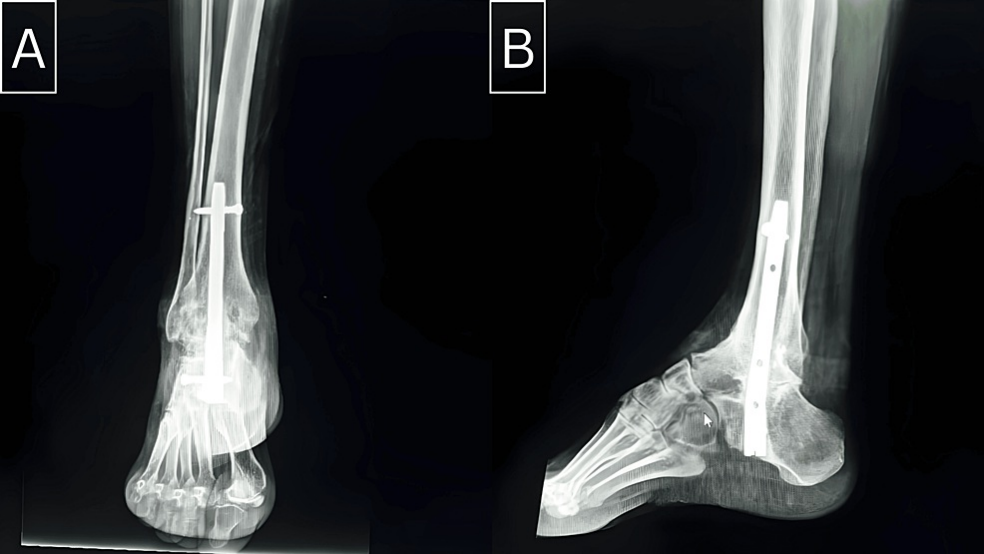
Weight-bearing anteroposterior (A) and lateral (B) X-ray of the ankle five months after tibio-talo-calcaneal arthrodesis with a retrograde femoral nail.
